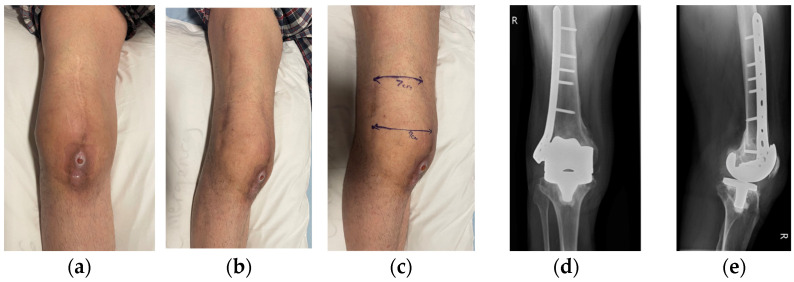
(a) Initial presentation of case 1 (anterior view). (b,c) Lateral view. (d) X-ray image from anterior view, and (e) X-ray image from lateral view.
